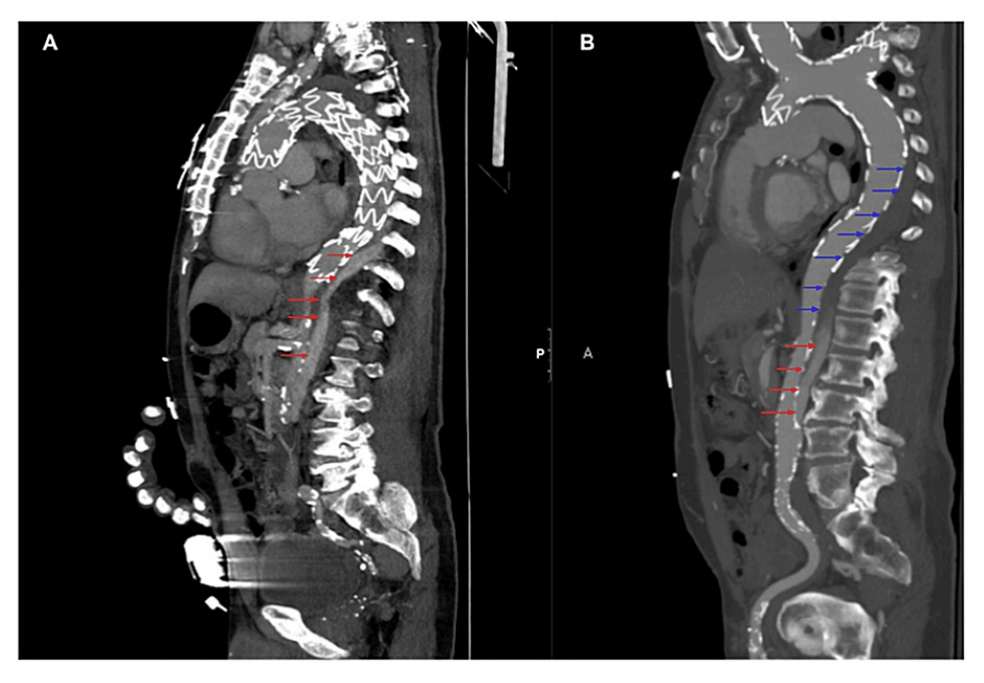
Aortic endoleak and subsequent endoleak progression and thrombosis (A) Computed tomography angiogram (CTA) of the chest/abdomen/pelvis demonstrating an endoleak with filling throughout the false lumen (red arrows) from a prior admission. (B) CTA chest/abdomen/pelvis from the patient’s presented admission showing further propagation of the endoleak compared to in A (red arrows). There is an absence of contrast within the dilated false lumen (blue arrows), suggesting interval thrombosis of a large portion of the endoleak from about T5-T8.
